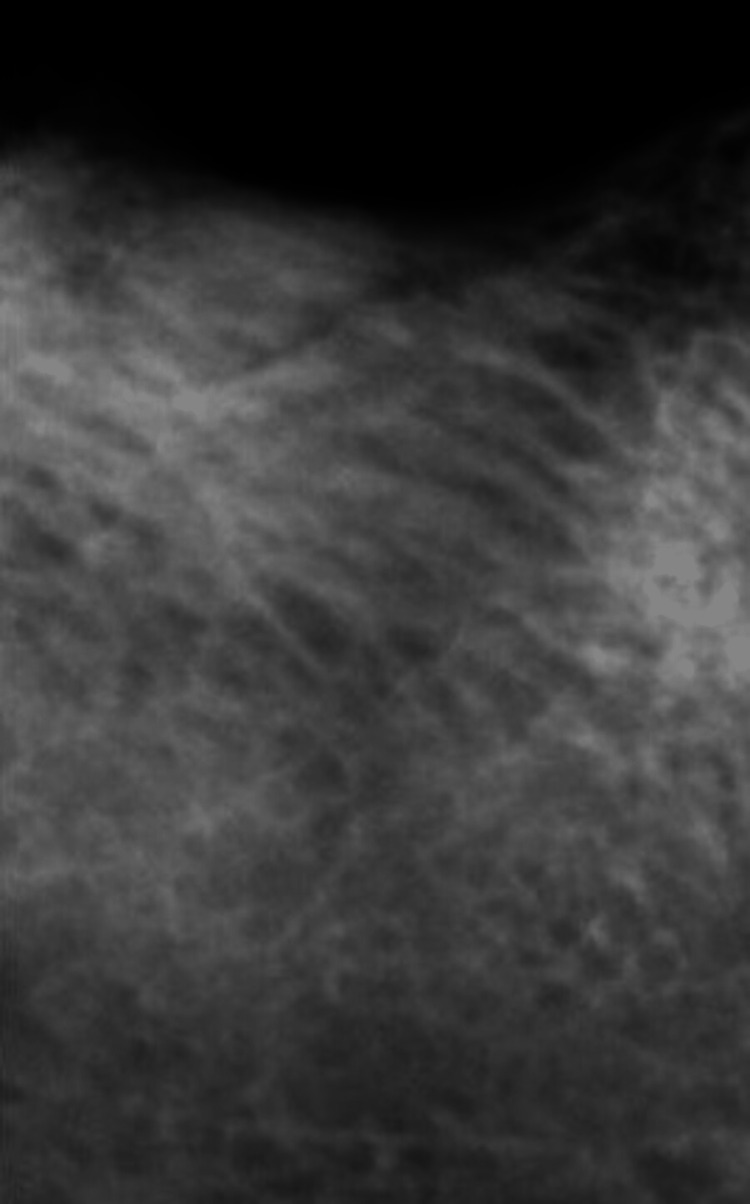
Sample radiographic image of a respondent presented to the examiners for bone quality type analysis.
